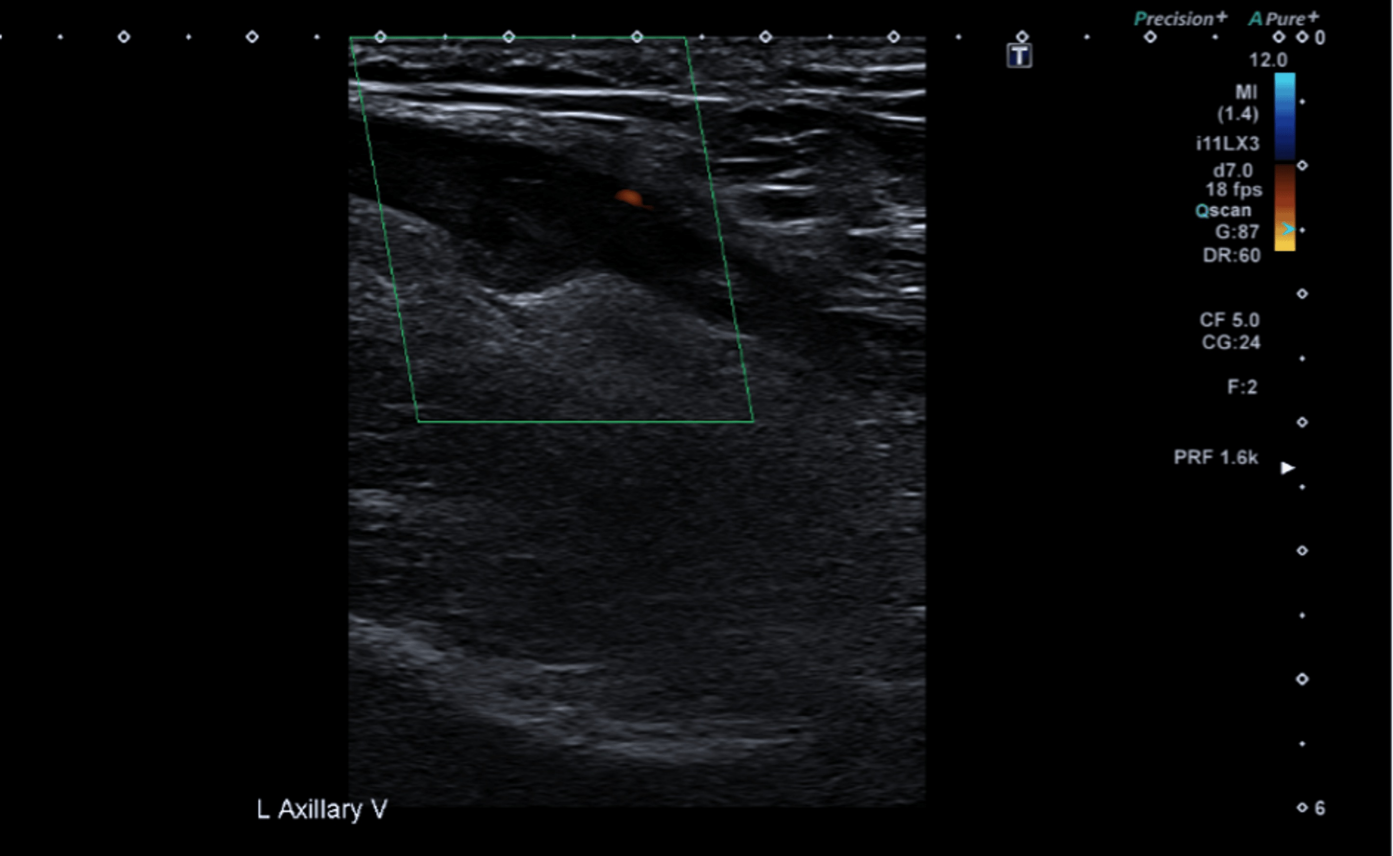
US shows no colour Doppler flow within the left axillary vein (green box) US: ultrasound
